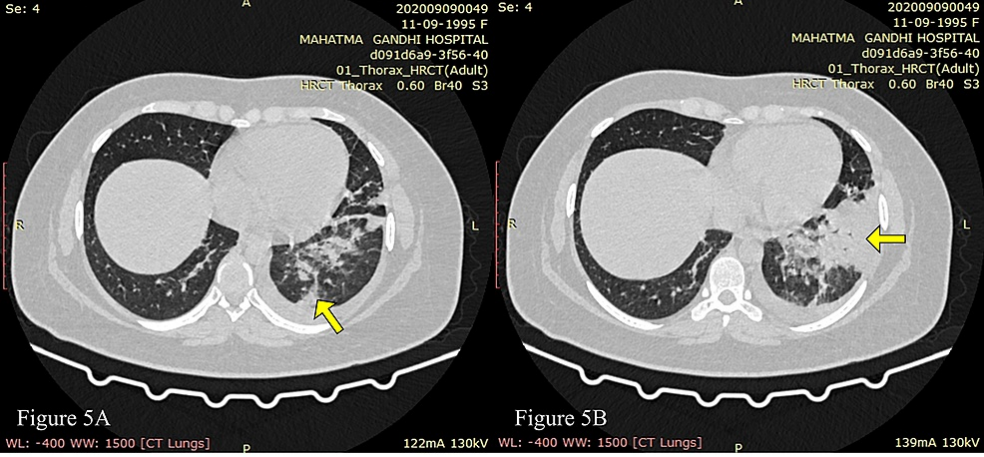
HRCT of the chest lung window (axial sections) showing ground-glass opacities and consolidation predominantly involving the left lower lobe of the lung (solid yellow arrows). CT severity score: 3/25. HRCT, high-resolution CT
